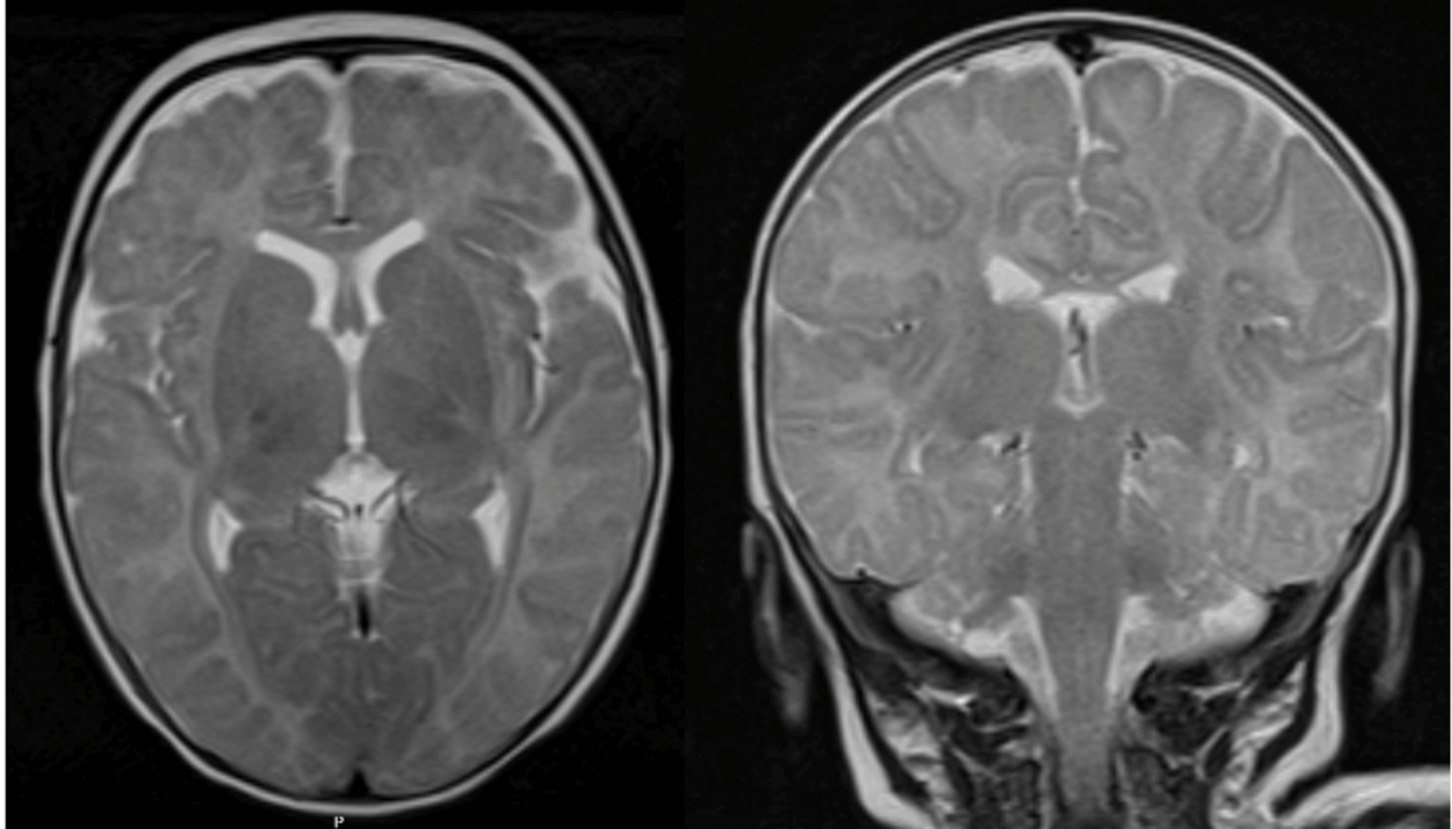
T2-weighted brain MRI - axial (left) and coronal (right).
